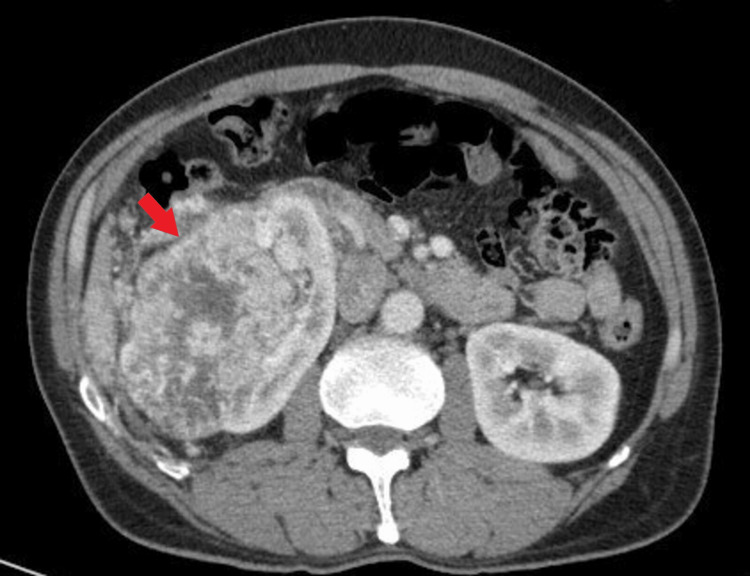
Initial CT scan of the abdomen and pelvis The red arrow is showing a large heterogenous right renal mass.
